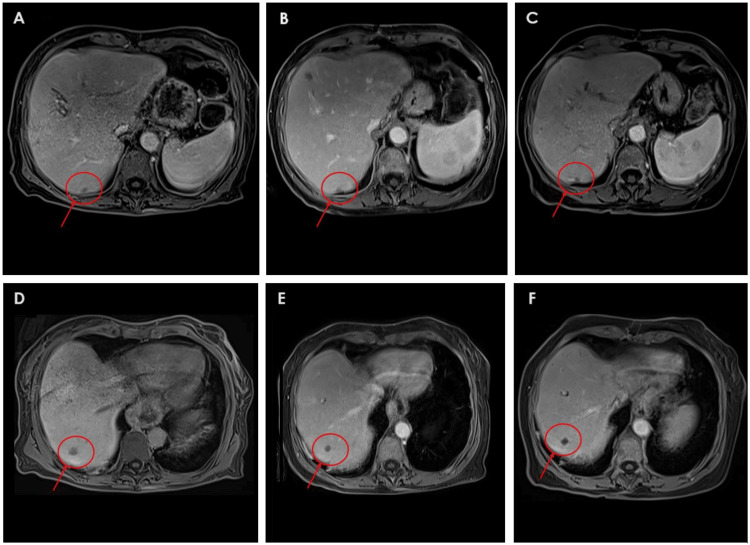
Sequential imaging of two hepatic metastases in segment VII during systemic treatment and before and after microwave ablation (A–C): Evolution of a subcapsular segment VII liver metastasis. (A) CE-MRI on April 23, 2024, shows an 11 mm hypointense hepatic metastatic lesion with low T1 signal intensity, intermediate T2 signal, and diffusion restriction, findings consistent with active disease. (B) CE-MRI on October 21, 2024, shows the lesion reduced to 6 mm, with intermediate T2 signal, low T1 signal, no diffusion restriction, and no contrast enhancement, again consistent with post-ablation changes and no signs of tumor viability. (C) CE-MRI on January 14, 2025, confirms a stable 6 mm treated lesion with no imaging evidence of recurrence. (D–F) Evolution of a second liver metastasis in segment VII. (D) CE-MRI on April 23, 2024, shows a 9 mm lesion (previously 13 mm) with low signal intensity on T1-weighted imaging and minimal contrast enhancement. (E) CE-MRI on October 21, 2024, reveals an 8 mm lesion with intermediate T2 signal, low T1 signal, no diffusion restriction, and no contrast enhancement, findings consistent with post-ablation changes and no evidence of tumor viability. (F) CE-MRI on January 14, 2025, shows a stable 8 mm lesion with identical signal characteristics, confirming the absence of tumor recurrence. CE-MRI: contrast-enhanced magnetic resonance imaging
